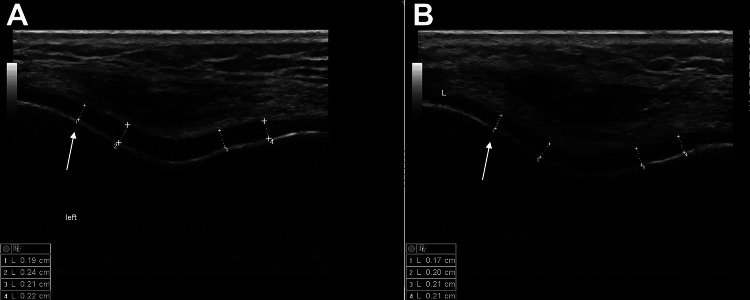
Ultrasound measurements A: Before the marathon. B: After the marathon. A significant decrease in femoral articular cartilage thickness can be seen at the left lateral femoral condyle (arrow).
